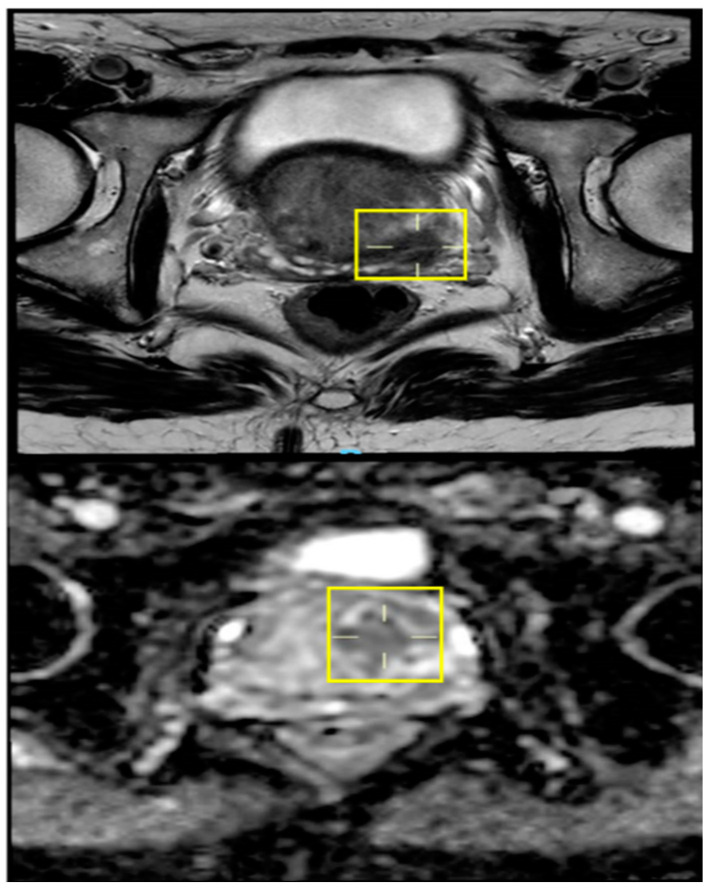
Images from the patient’s MRI with the colon cancer metastasis to the area of the prostate (yellow squares).
